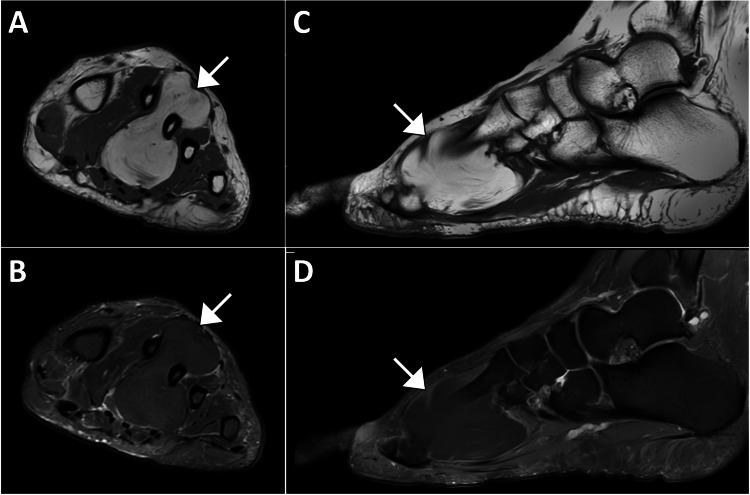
Magnetic resonance imaging of the left foot (Panel A shows a coronal T1-weighted image without fat saturation, Panel B shows a coronal T1-weighted image with fat saturation, Panel C shows a sagittal T1-weighted image without fat saturation, and Panel D shows a sagittal T1-weighted image with fat saturation) showing a large bilobed intramuscular lesion with both plantar and dorsal components, measuring 4.3 cm in anterior-posterior diameter, 2.5 cm transversely, and 4.5 cm longitudinally. The lesion demonstrates fat signal intensity on all sequences, with complete suppression on fat-saturated images. Likely originating from the dorsal interosseous muscle, the lesion decompresses through the second webspace to the plantar side, with potential involvement of the adductor hallucis longus muscle.
